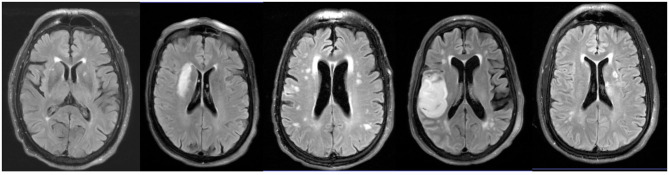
WMH location in AIS patients with moderate to severe WMH. Representative axial FLAIR images of WMH location in AIS patients enrolled in this study.
